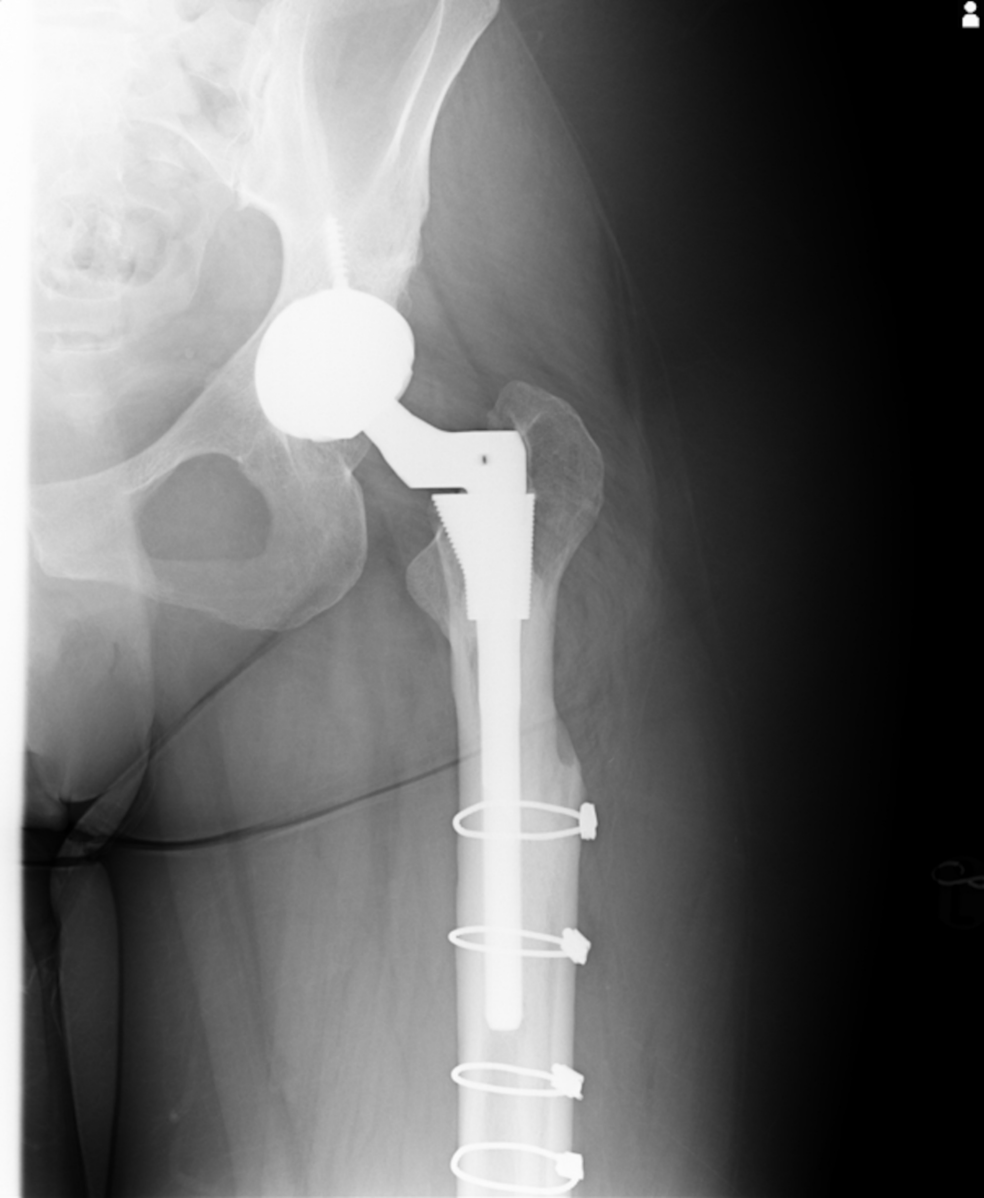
AP radiograph one year post-operatively of the left femur cortical strut allograft. AP, anteroposterior
